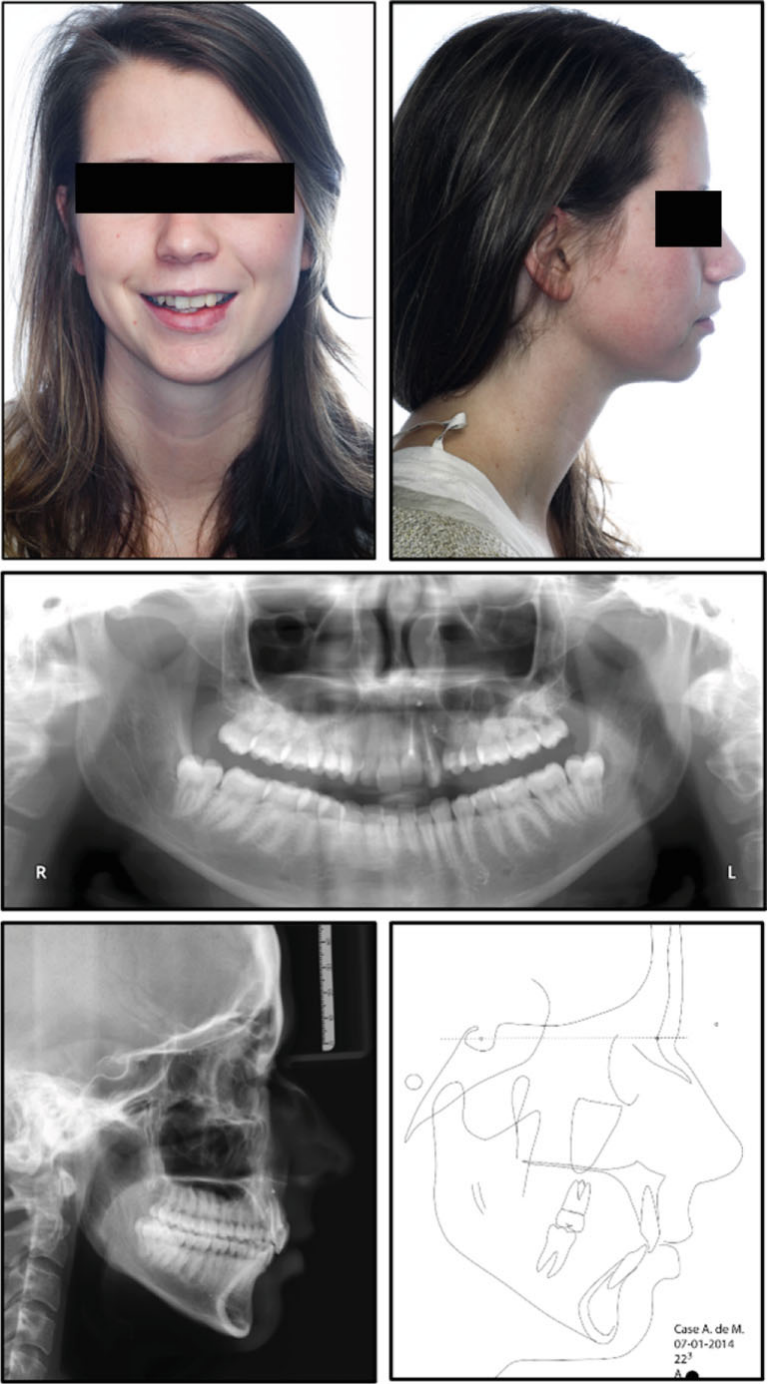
Facial analysis, panoramic and lateral radiographs, and cephalometry before treatment start.
